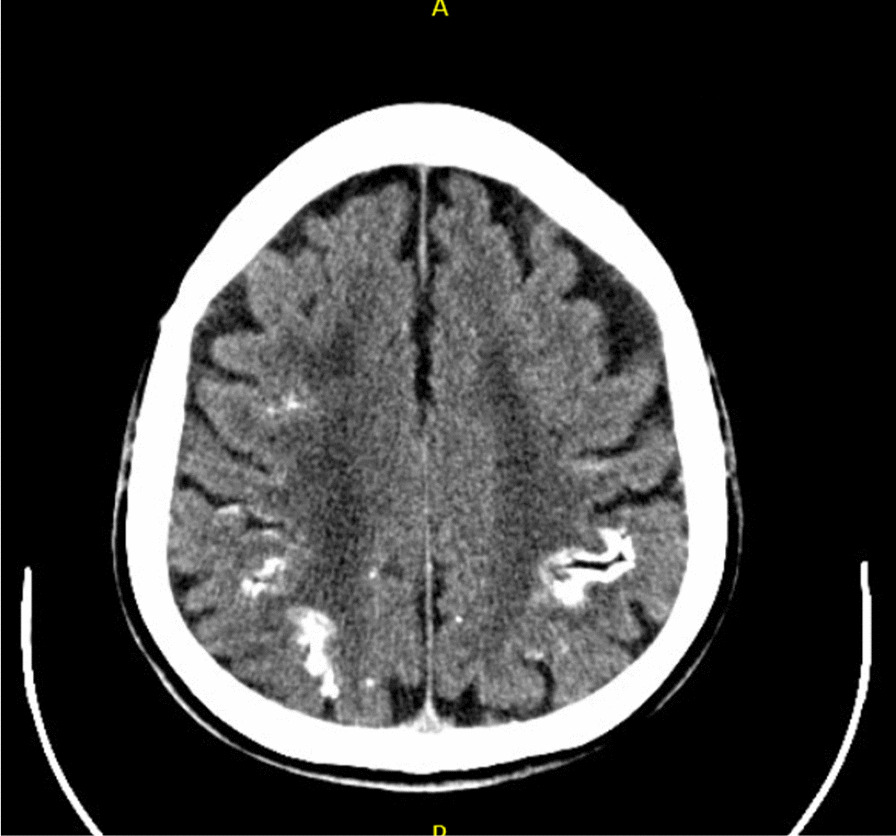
Head CT scan showing diffuse brain atrophy with cortical calcifications
